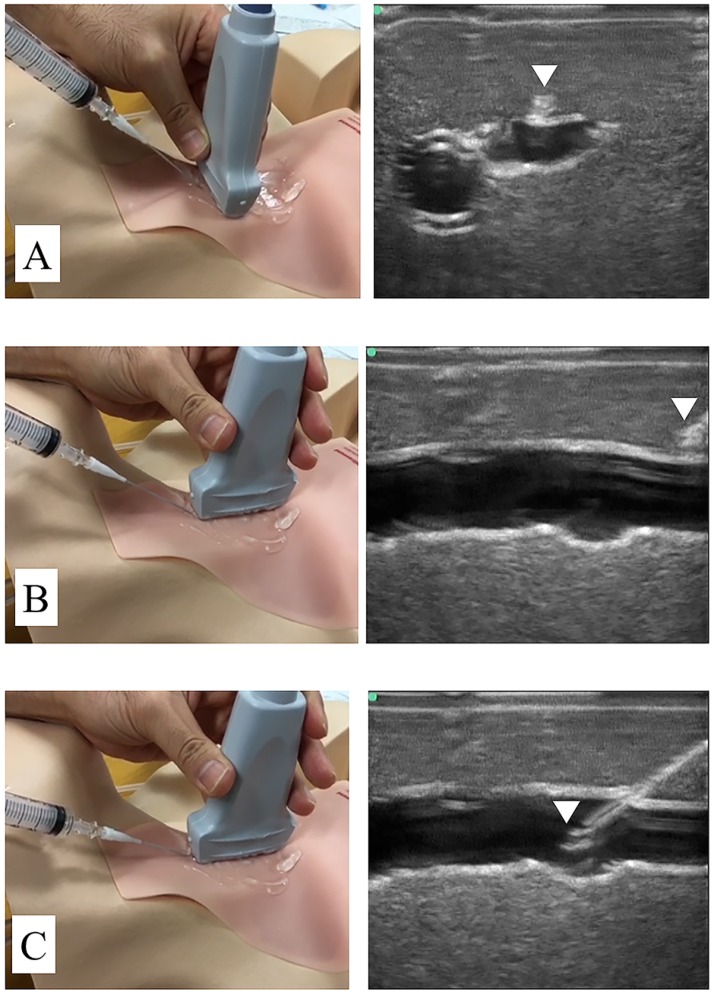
The needle as visualized on ultrasonography. A) The needle tip (arrow) is visualized as a dot between the skin and anterior wall of the target vein in the short-axis view. B) After rotating the transducer by 90°, the entire length of the needle (arrow) is observed in the long-axis view. C) The needle (arrow) is observed puncturing the anterior wall of the target vein.
